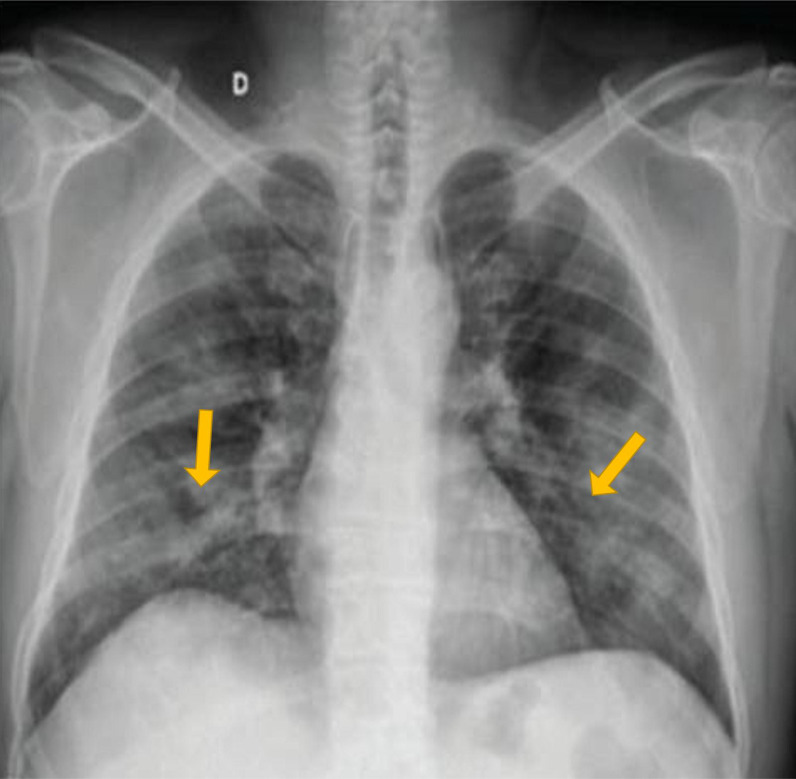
Grade 2 chest X-ray: bilateral alveolar consolidation affecting the whole lung lobe (pan-lobar consolidation) or widespread severe findings.
